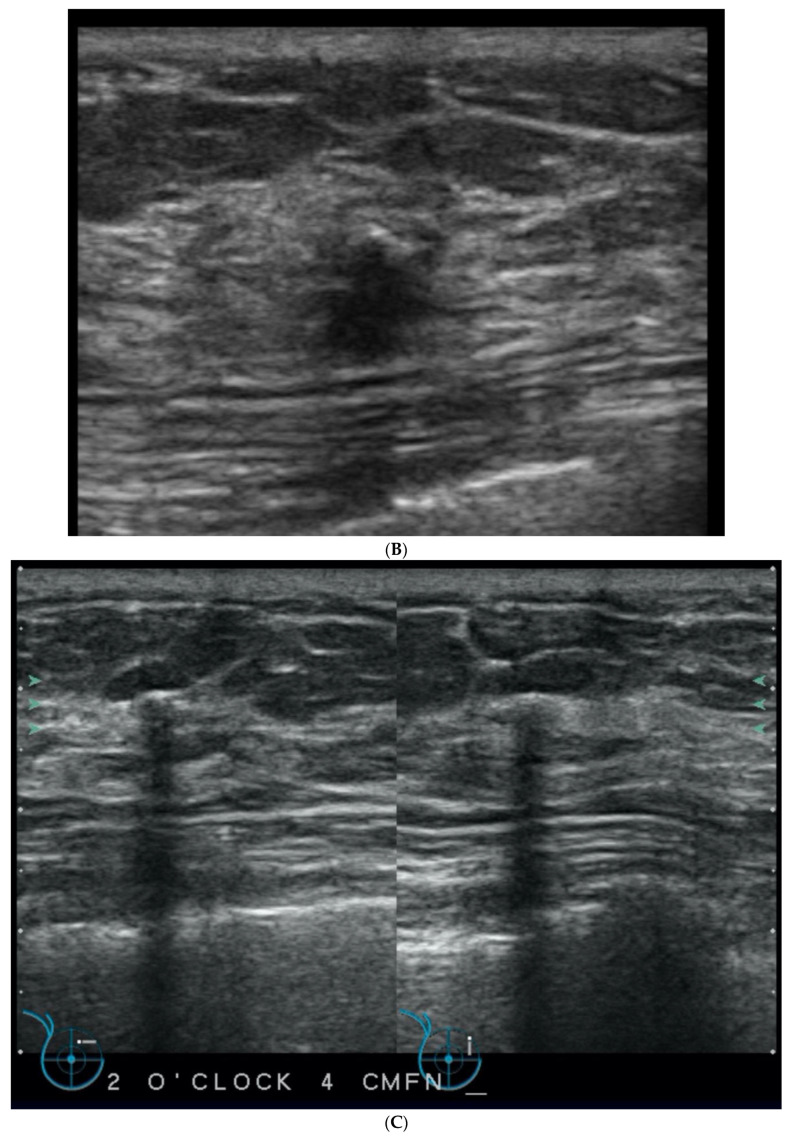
(A) Negative screening mammogram in a 73-year-old woman with category C density. Screening ultrasound showed masses in (B) left and (C) right breasts. Tomosynthesis is not part of the screening mammography program, but was performed after masses were found on US, but was also negative (not shown). Mass on left showed invasive lobular carcinoma on core biopsy. Mass on right showed atypical lobular hyperplasia.
